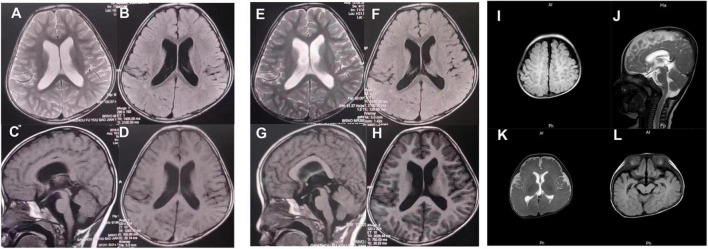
At the age of 4 years, 6 months (A–D), and 5 years 1 month (E–H), brain MRI (3T) of patient 1 showed lateral ventricles dilation, dilation of Virchow-Robin spaces, and dilated the third ventricle. (I–L) Brain MRI (3T) of patient 3 revealed delayed myelination and a widened subarachnoid space.
